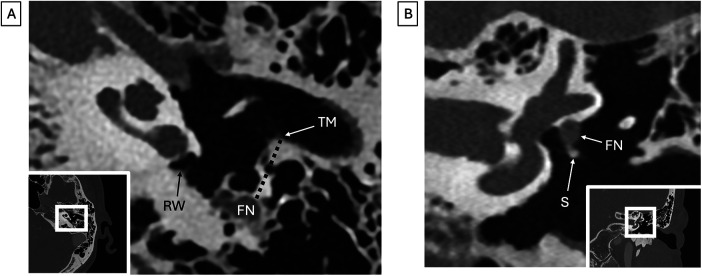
Facial nerve anatomy (both images are from the same patient). (A) Photon counting axial CT shows a normal facial recess width (dotted line), measured from the mastoid segment of the facial nerve (FN) to the annulus of the tympanic membrane (TM) at the level of the round window (RW). (B) Overhanging facial nerve. Photon counting coronal CT image shows dehiscence of the facial nerve canal with extension of the tympanic segment of the facial nerve (FN) into the tympanic cavity, with contact of the stapes (S).
